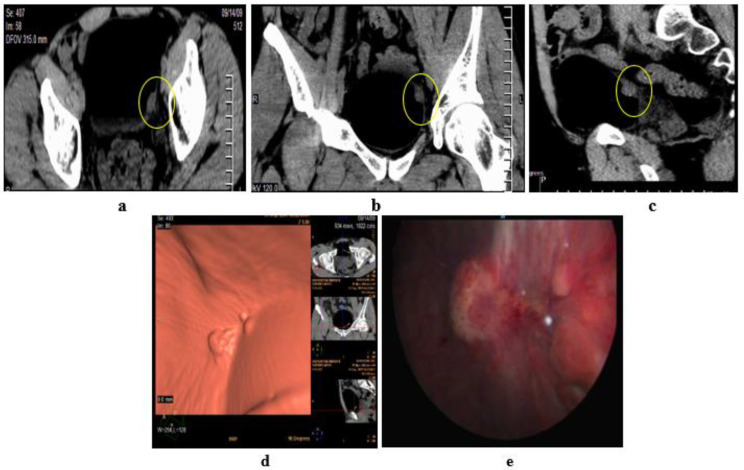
Papillary urothelial cell carcinoma of the bladder. (a–c) Axial, coronal, sagittal images: only one lesion at the left lateral bladder wall. (d) CT virtual cystoscopic volume rendering image: 3 small lesions at the left lateral bladder wall. (e) Conventional cystoscopic image confirms the presence of the three lesions at the left lateral bladder wall [42].
